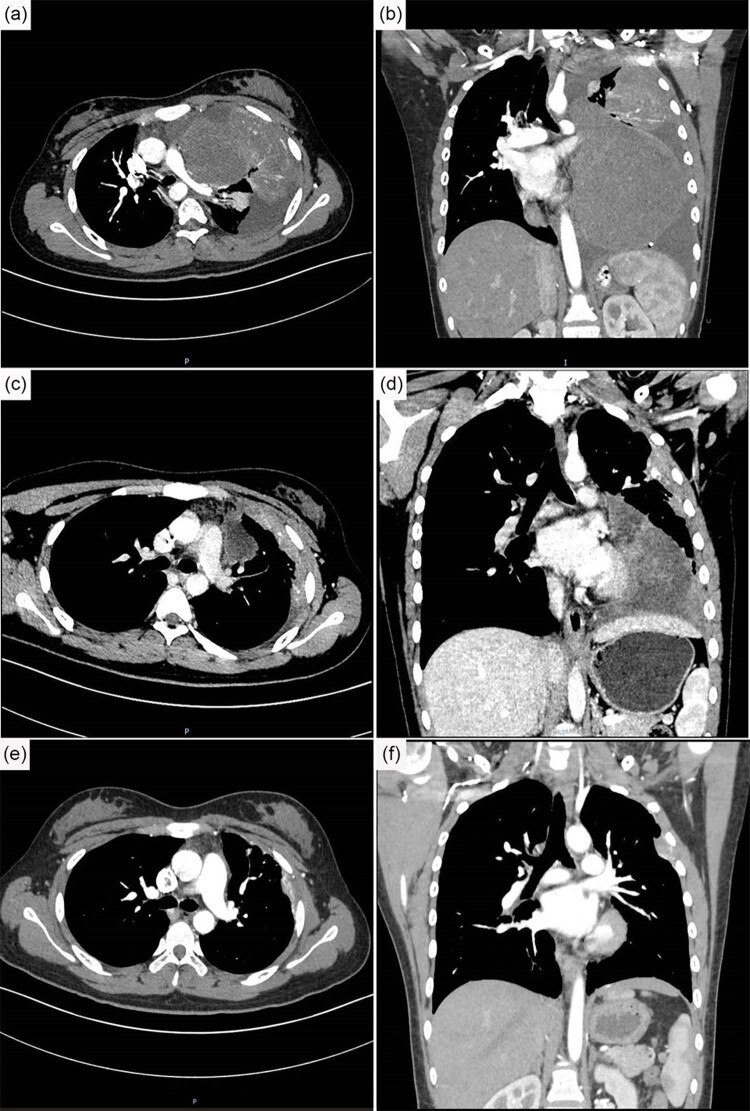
CT scan of the thorax. (a and b) A mass with multiple small vessel shadows was observed near the mediastinum. A pleural effusion was found in the left thorax. (c and d) Chest CT after one cycle of chemotherapy showed that the mass and fluid decreased. (e and f) After six treatment cycles, the tumor was hard to observe, and no pleural effusion could be observed. CT: computed tomography.
